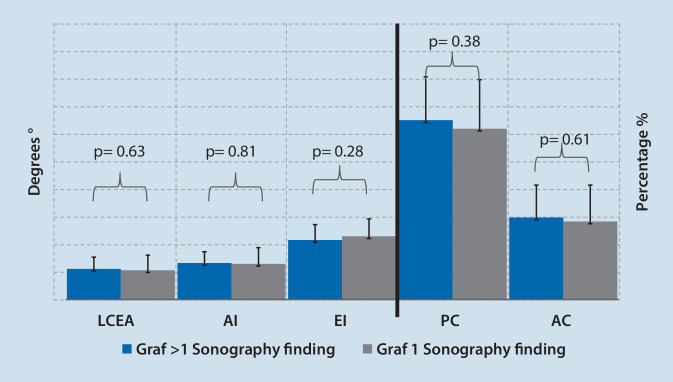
Comparison of radiological parameters in the mature pelvis in AP projection showed no significant difference between patients presenting with sonographic hip development Graf 1 or Graf > 1 in U3 examination. *LCEA* lateral center edge angle, *AI* acetabular index, *EI* extrusion index, *PC* posterior coverage, *AC* anterior coverage
